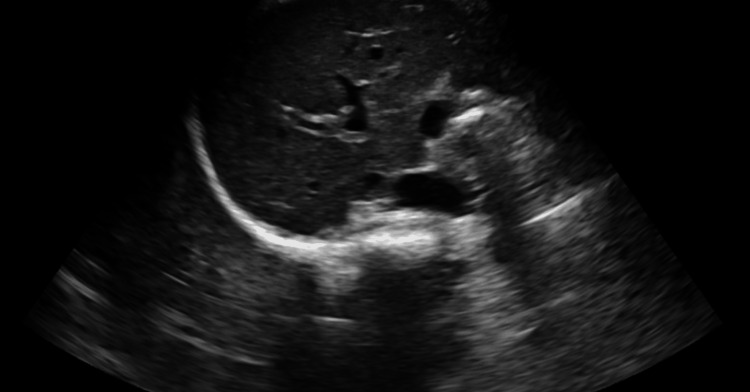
Abdominal ultrasound demonstrating mild gallbladder wall thickening; nonspecific, and no gallstones.
